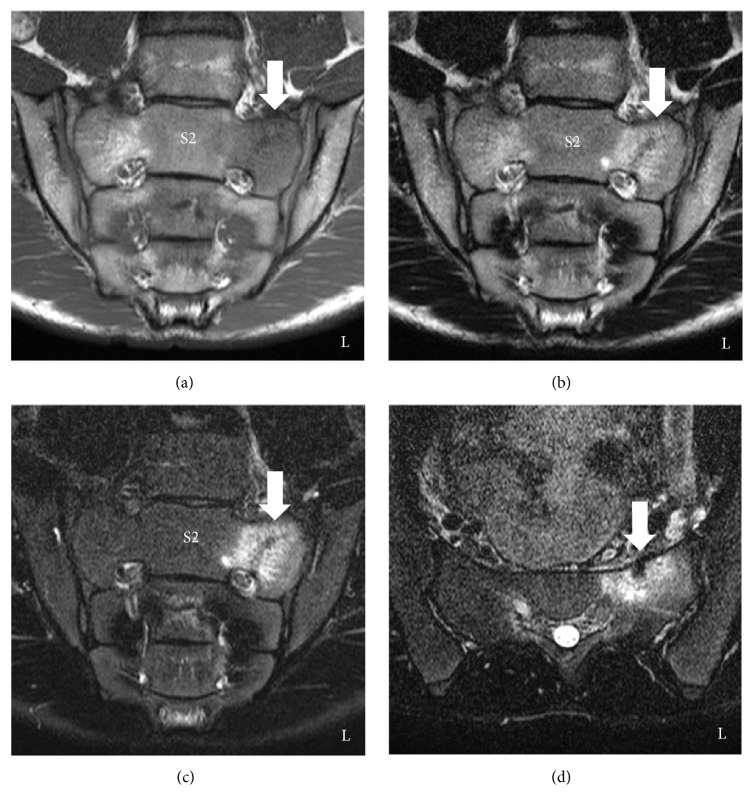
Magnetic resonance image of the sacrum at the first visit to our hospital. (a) Coronal plane on a T1-weighted image. (b) Coronal plane on a T2-weighted image. (c) Coronal plane on a short TI inversion recovery (STIR) image. (d) Axial plane at the S2 level on a STIR image. The left sacral ala on a T1-weighted image shows a low intensity (a), a T2-weighted image shows a high intensity (b), and on a STIR image shows a high intensity (c) compared with the right sacral ala. These radiological findings indicated bone marrow edema. Oblique lineal signal void (white arrows) can be seen in the bone marrow edema; these are fracture lines. An axial plane on a STIR image shows a low-intensity area (white arrow) surrounded by a high-intensity area in the left ventral surface of the sacral ala (d).
